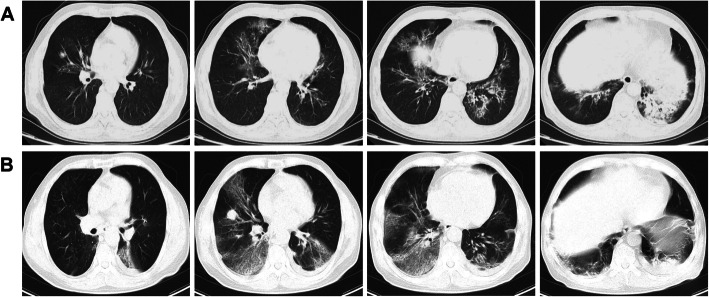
CT scan of the lung. a On the 1st day, a CT scan showed bronchitis and inflammation in the right lung lobes, the left ligule lobe and the left lower lobe. b: On the 9th day, a CT scan showed that some of the inflammation was alleviated, but inflammation in most lung lobes was worse
